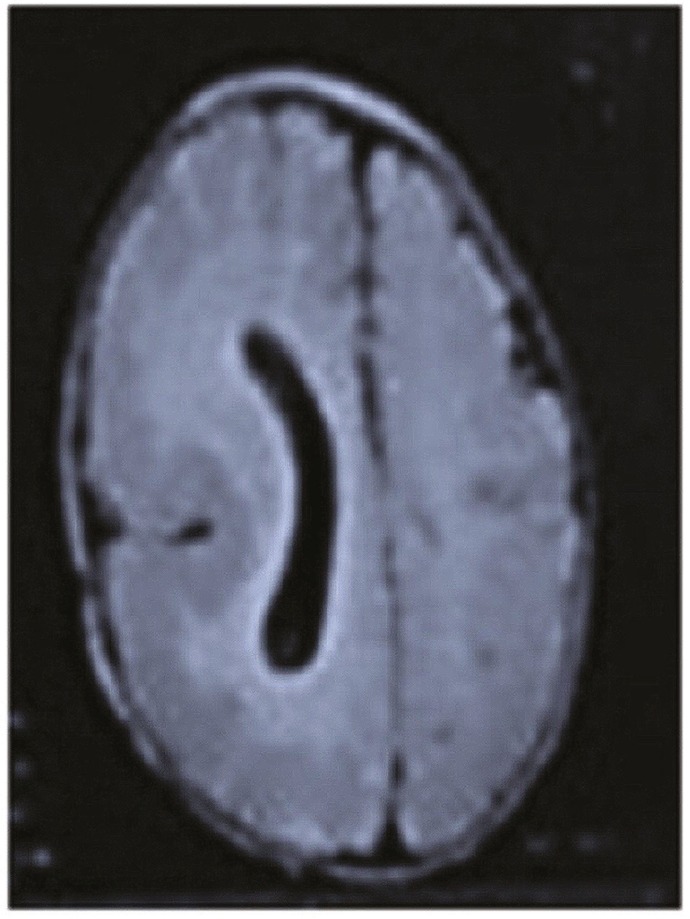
MRI showing hemimegaleencephaly
